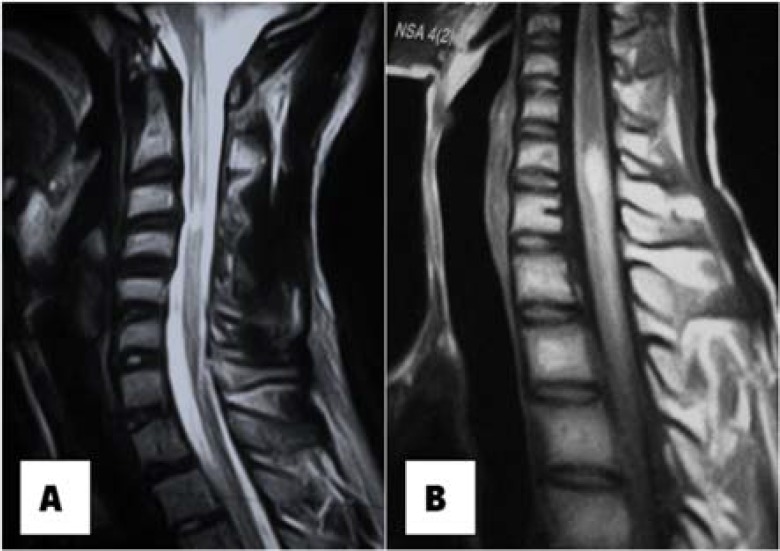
Magnetic resonance imaging (MRI) in T1 sequence with injection, carried out after 1 month of symptomatology, objective a hypersignal opposite C1-C5 (A), MRI in T2 sequence without injection performed after 2 months of its symptomatology, objective a hypersignal opposite C3-C6 (B)
